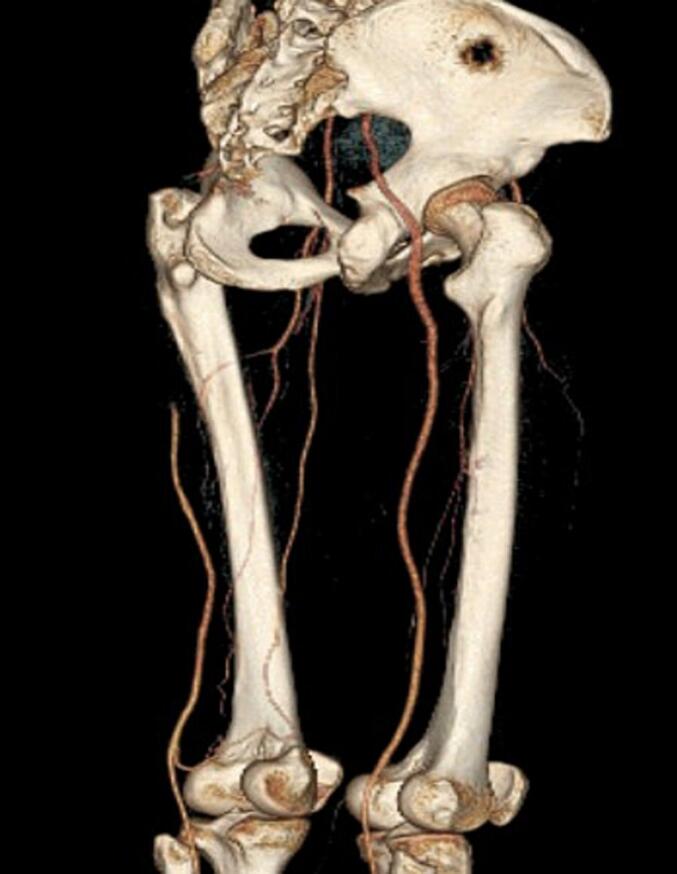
Posterior lateral view 3D CT reconstruction.
